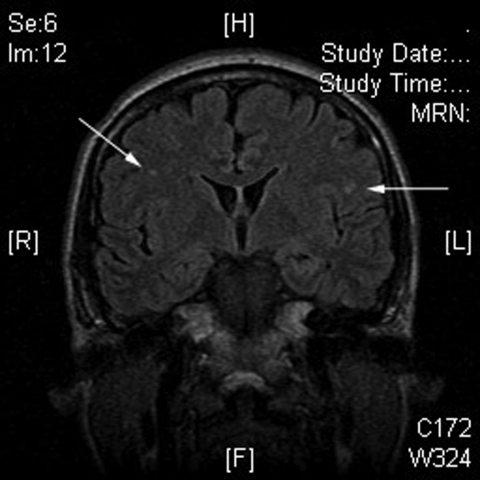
Magnetic resonance imaging of the brain of patient B, showing several nonspecific areas of enhancement (arrows), which suggests encephalitis, given the clinical scenario.
